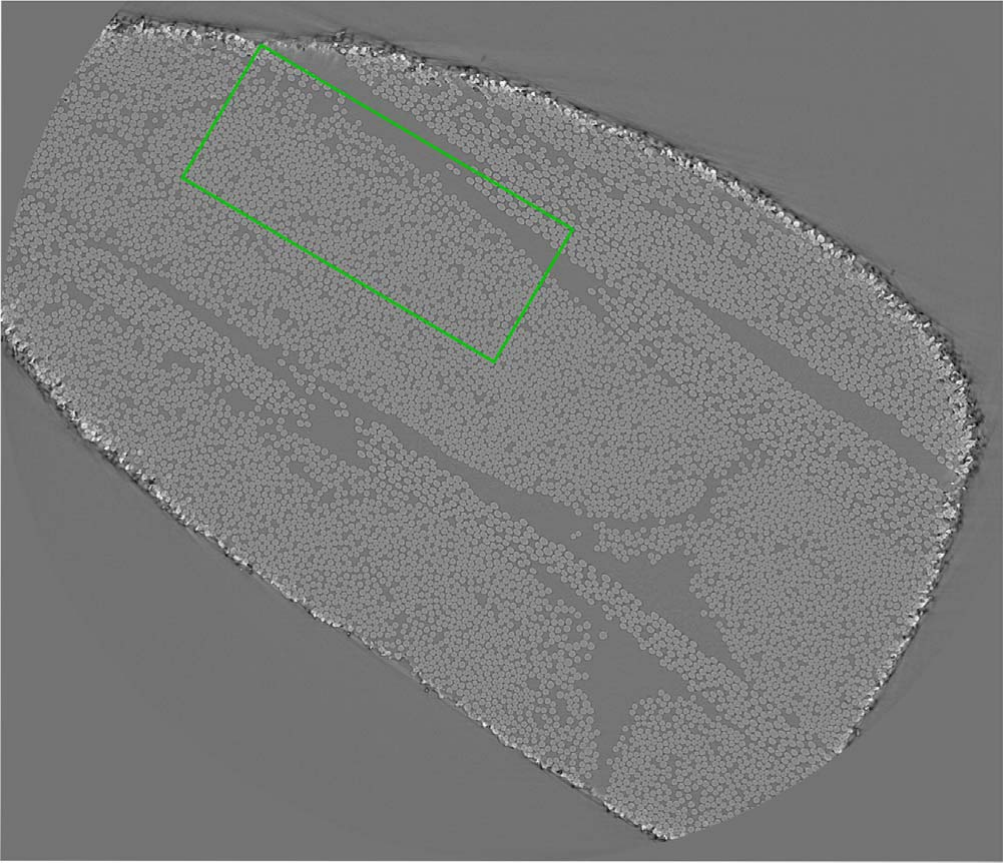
X-ray CT cross-sectional slice for the synchrotron scan with the sample area of great overlap between all six scans indicated in *green*.
